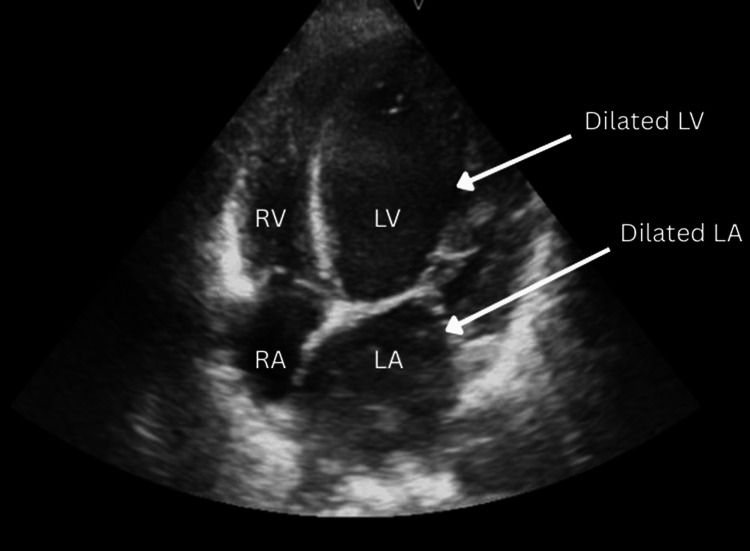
Transthoracic echocardiography (apical four-chamber view) - image 1 Two-dimensional image demonstrating global left ventricular hypokinesia with severe left ventricular grade II diastolic dysfunction and dilated left ventricle with grade II mitral regurgitation; mild (grade I) tricuspid regurgitation was also noted RV: right ventricle; RA: right atrium; LV: left ventricle; LA: left atrium
